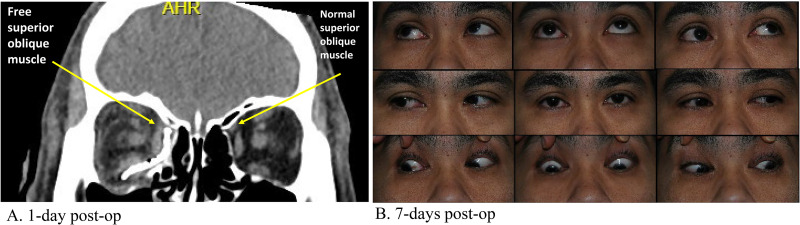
Post-operative CT facial bones (coronal view) (A) and orthoptic assessment (B) following hardware removal and revision of ORIF. The metallic mesh no longer appears to be impinging on the superior oblique muscle of the right eye (arrow) (A). Photos of the nine cardinal positions of gaze demonstrates improved alignment in primary position and an increased ability of the right eye to elevate in adduction (B).
